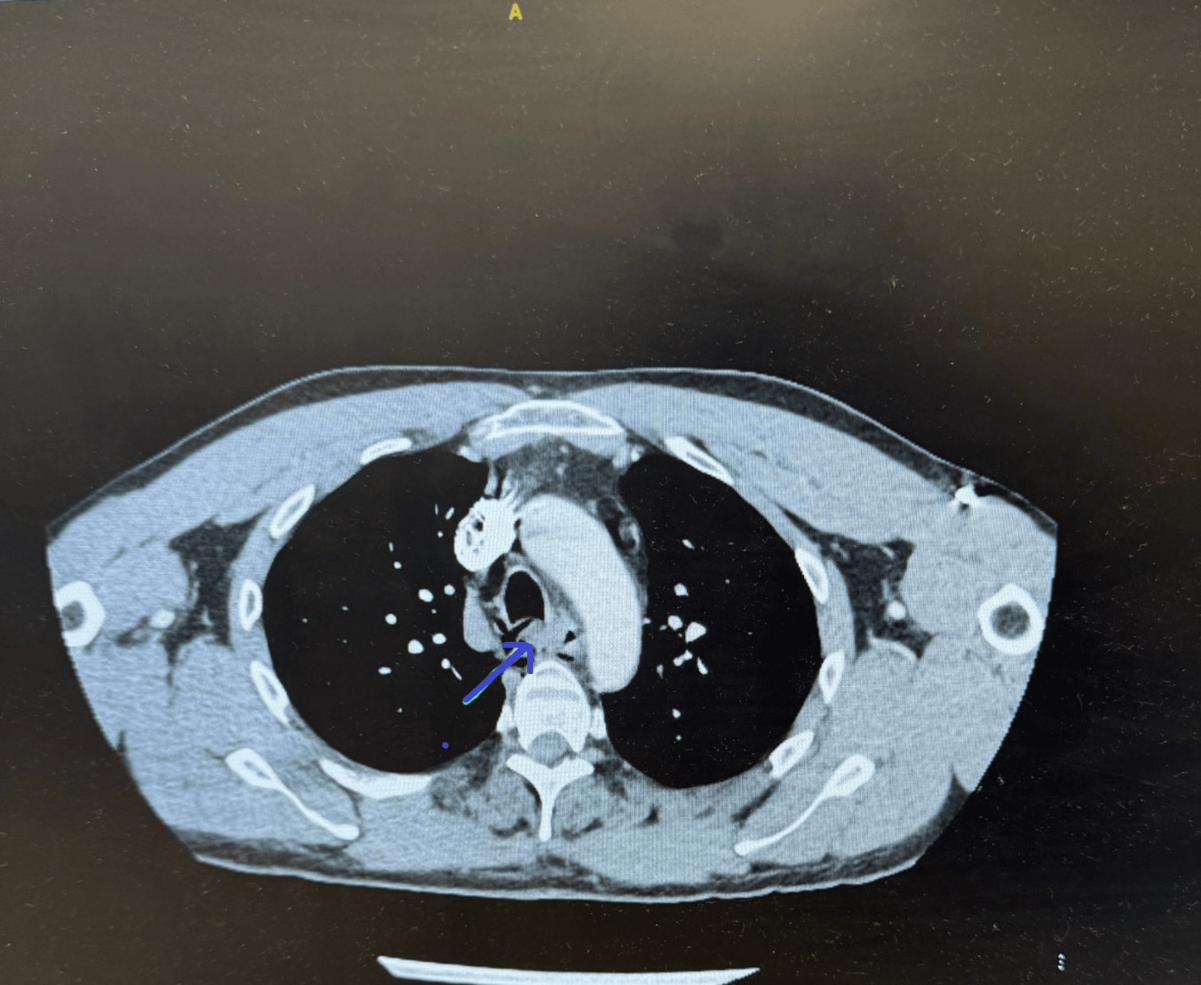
CT of the neck with contrast showing esophageal blockade with rupture
